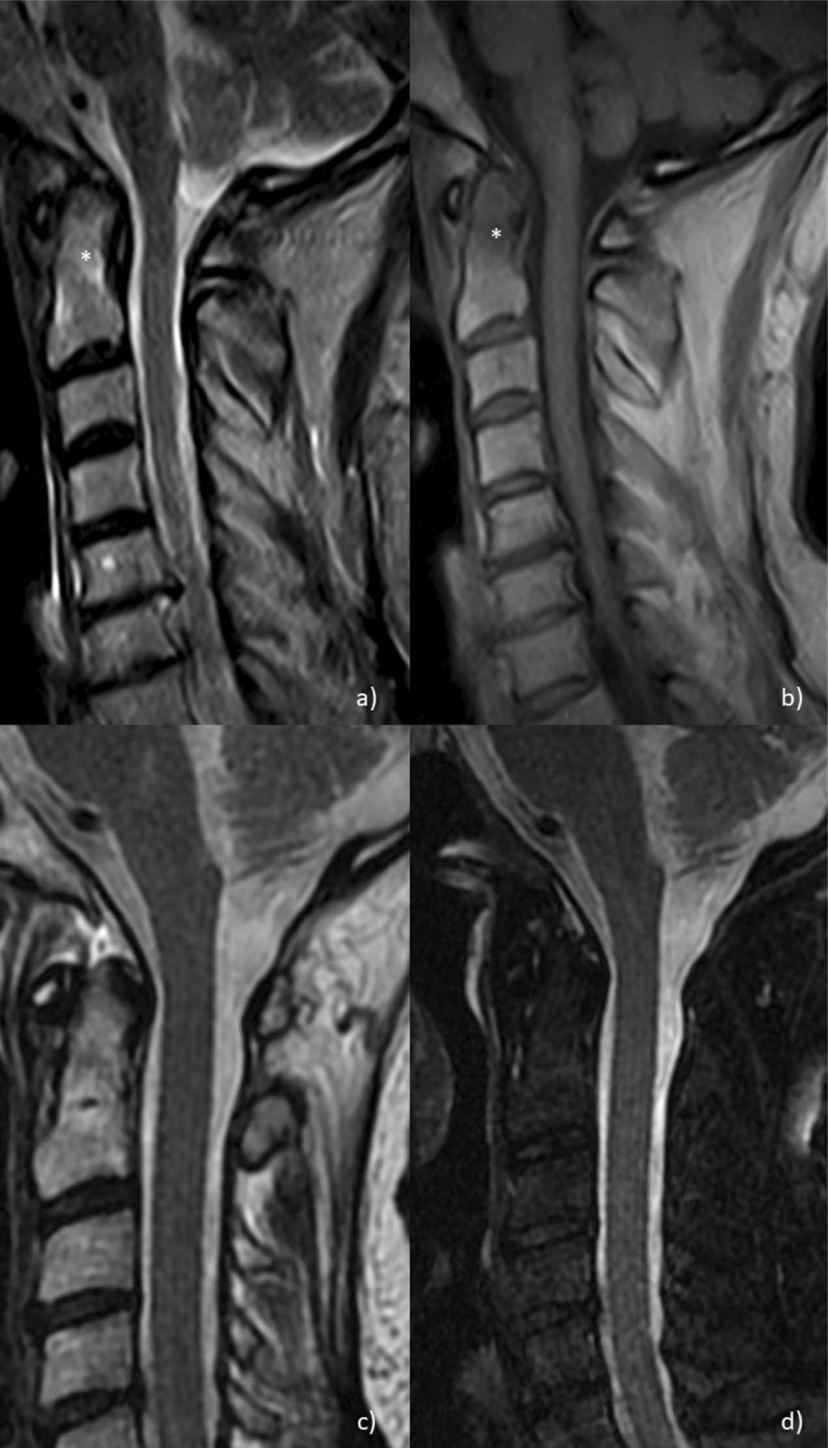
Sagittal T2 (a) and sagittal T1 (b) MRI images showing slight bone marrow edema of the odontoid peg (asterisk). Sagittal T2 (c), sagittal fat-saturated T2 (d) MRI images showing normal imaging findings at the level of the craniocervical junction.
